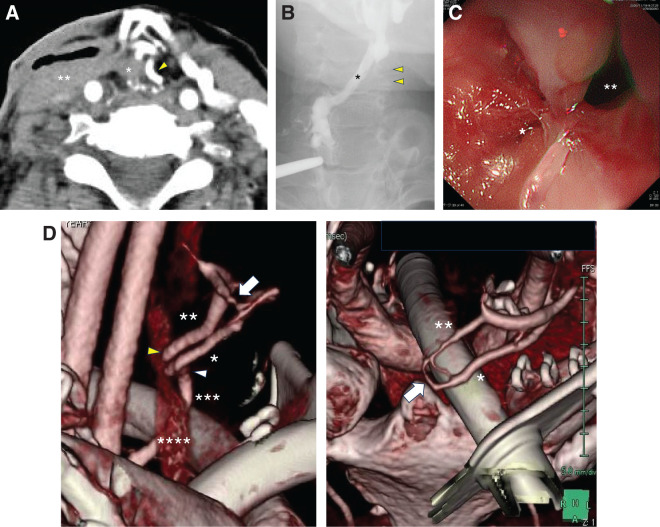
(A) Contrast-enhanced CT image at 7 months after surgery. The asterisk indicates the esophagus. The double asterisk indicates subcutaneous abscess. The yellow arrowhead indicates the third jejunal artery. (B) Fluoroscopy at 7 months after surgery. The asterisk indicates fistula at the pharyngealjejunal anastomosis with massive leakage. Yellow arrowheads indicate estimated location of the free jejunal graft. (C) Endoscopic observation revealed a complete obstruction due to scarring at the pharyngealjejunal anastomosis (asterisk) and a fistula opening on its side (double asterisk). (D) 3D reconstruction of a postoperative contrast-enhanced CT obtained on POD11. The single asterisk indicates the third jejunal artery. The double asterisk indicates the third jejunal vein. The triple asterisk indicates the left transverse cervical artery. The quadruple asterisk indicates the left internal jugular vein. The white arrowhead indicates vascular anastomosis between the second jejunal artery and the left transverse cervical artery. The yellow arrowhead indicates vascular anastomosis between the second jejunal vein and the left internal juggler vein. White arrows indicate the AVF in the free jejunal mesentery.
